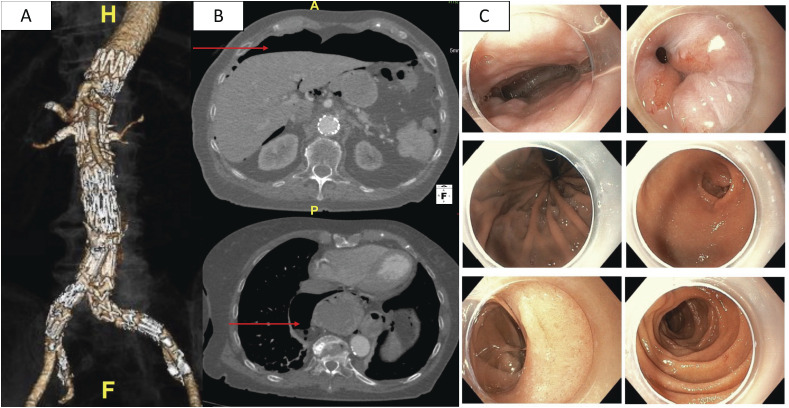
Panel A:* 3D reconstruction of post-op CTA images, were no endoleak and a successful target vessel patency was revealed. *Panel B:* Presence of free air in peritoneum and in mediastinum (red arrows). *Panel C:* Images of intraoperative upper gastrointestinal endoscopy, confirming absence of any perforation sites
